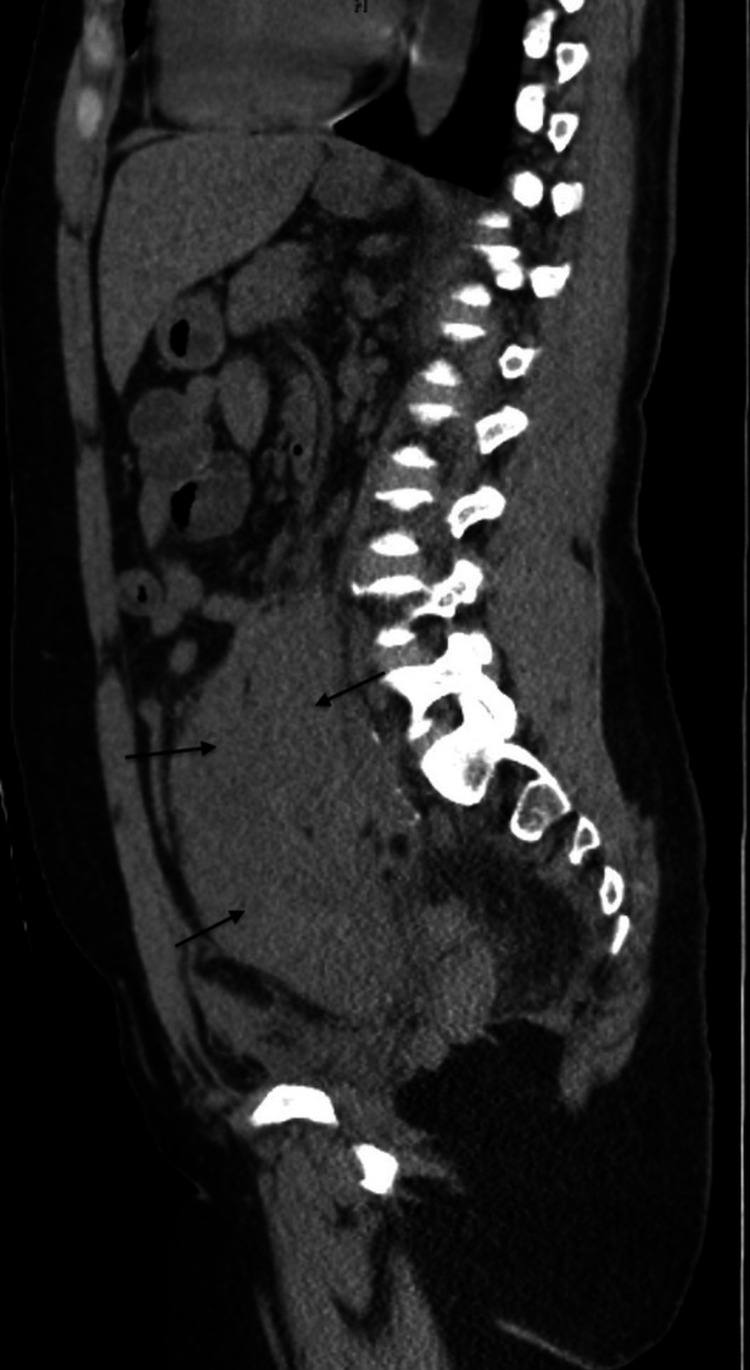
CT sagital image Retroperitoneal hematoma " arrows"
